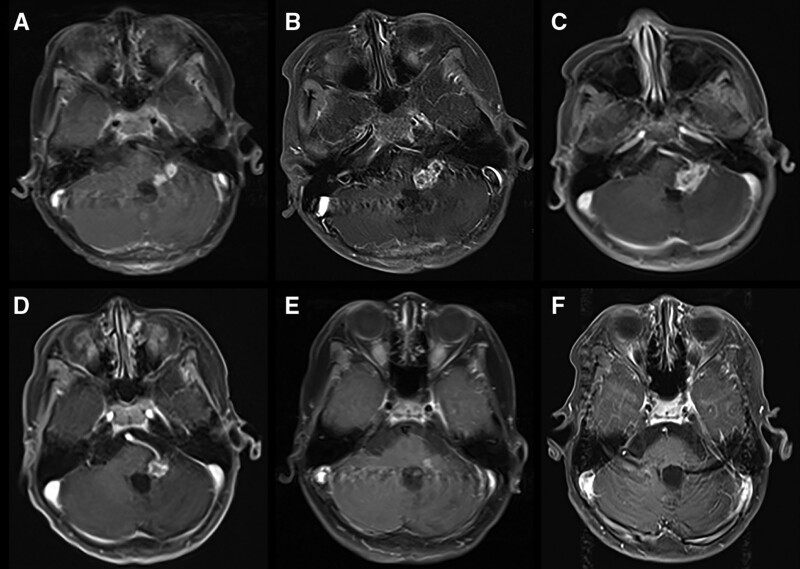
Chronological changes on magnetic resonance imaging (MRI) show second tumor recurrence and response. Notes: Coronal MRI of T1 contrast-enhanced axial images were taken at the following times: (A) diagnosis of relapse of the second tumor, (B) after 2 cycles of EP, (C) after 1 cycle of TMZ + DDP, (D) after 5 months of TMZ + apatinib, (E) after 7 months of TMZ + apatinib, and (F) after 9 months of TMZ + apatinib. Abbreviations: EP = etopside + cisplatin, TMZ + DDP = TMZ + cisplatin, MRI = magnetic resonance imaging, EPNs = Ependymomas, CNS = central nervous system, rEPNs = recurrent ependymomas, EANO = European Association of Neuro-Oncology, CR = complete response, VEGF = vascular endothelial growth factor, VEGFRs = vascular endothelial growth factor receptors, TKI = tyrosine kinase inhibitor, TMZ = temozolomide, PFS = progression-free survival, OS = overall survival.
